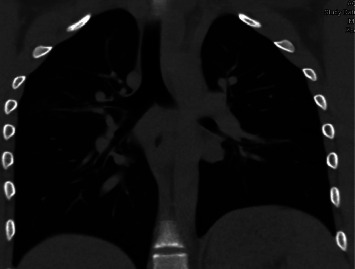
Post-extubation coronal view of CT chest scan showing the resolution of ground-glass opacities following diuretic therapy.
